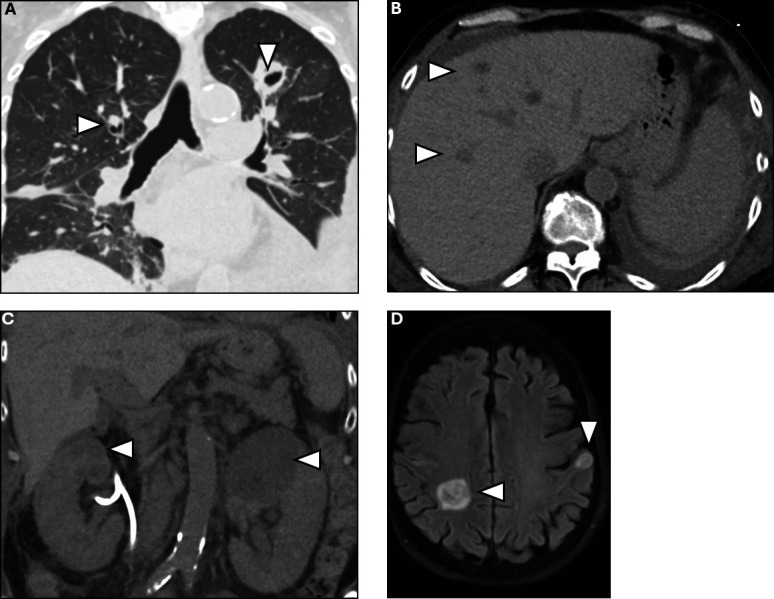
Non-contrast CT of the chest, abdomen, and pelvis demonstrating bilateral cavitary nodules (A), hepatic hypodensities (B), and bilateral kidney hypodensities (C) suggestive of abscesses. T2 FLAIR MRI brain with and without contrast demonstrating right parietal lobe and left temporal lobe hyperintensities consistent with septic emboli (D).
